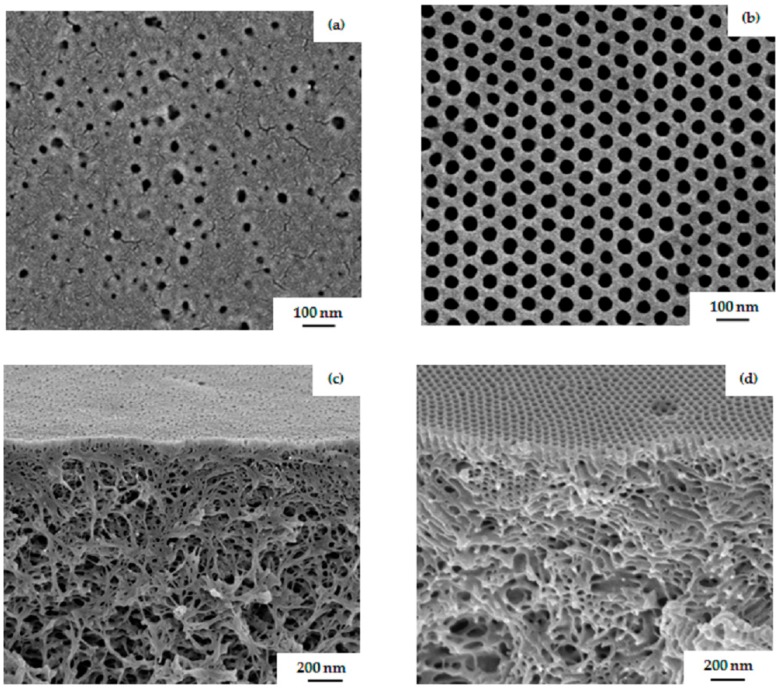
SEM topography images of membranes prepared from (a) 23 wt % PS_76_-PSMA_24_^135^ and (b) 23 wt % PS_81_-PGMA_19_^128^ in THF/DMF 50/50 wt %. The corresponding cross-section views for each case are shown in (c) and (d) images, respectively. The time of evaporation was 10 s.
